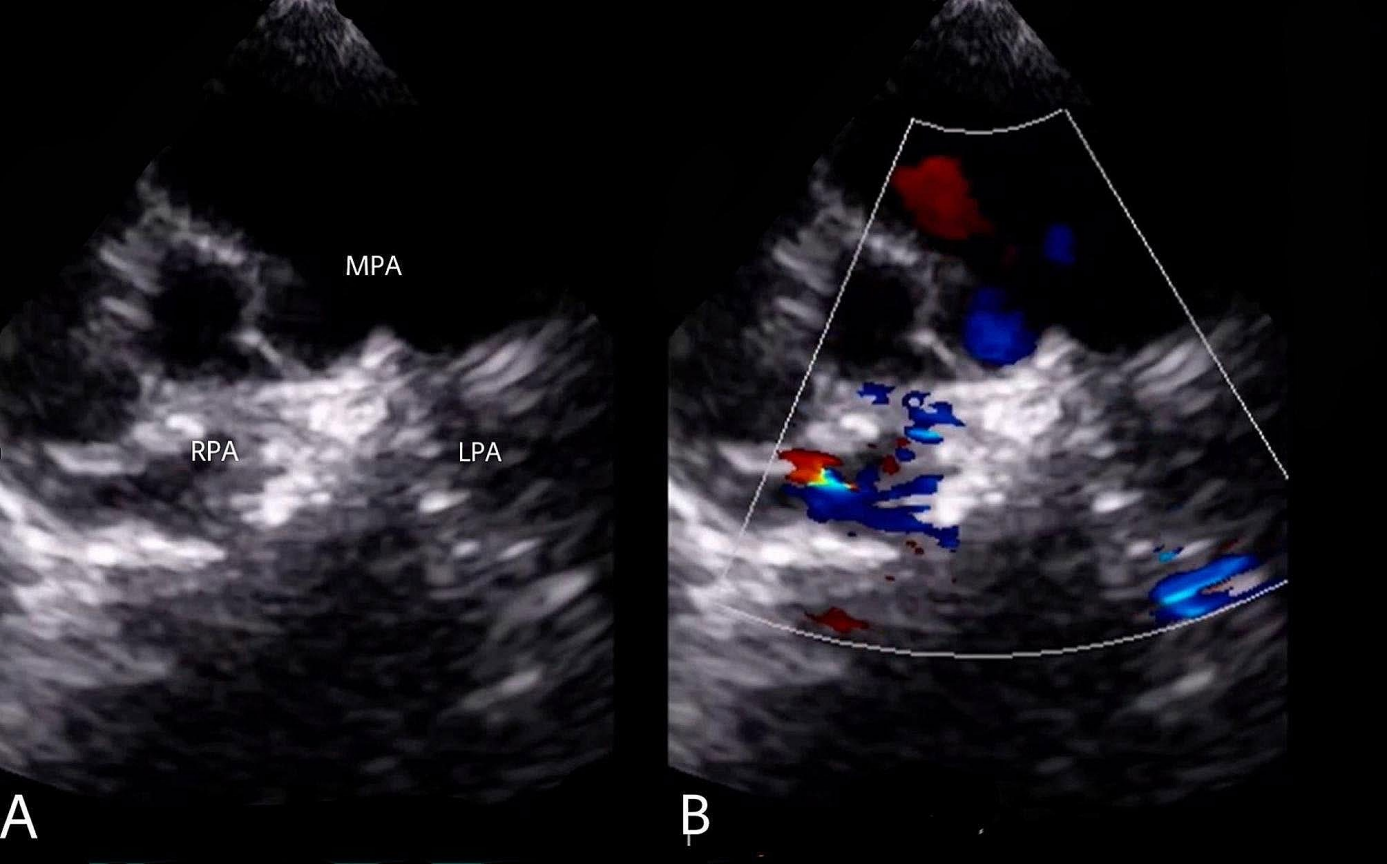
Preoperative transthoracic echocardiography. Preoperative transthoracic echocardiography (short axis view): (A) demonstrating the anatomy of the pulmonary arteries with an enlarged main pulmonary artery with small, tortuous and hypoplastic branch pulmonary arteries. (B) color flow doppler demonstrated that the left pulmonary artery has a peak gradient of 73 mmHg with a peak velocity of 4.3 m/s and the right pulmonary artery having a peak gradient of 46 mmHg with a peak velocity of 3.4 m/s. *(MPA: main pulmonary artery, RPA: right pulmonary artery, LPA: left pulmonary artery)* (see supplementary video 1)
